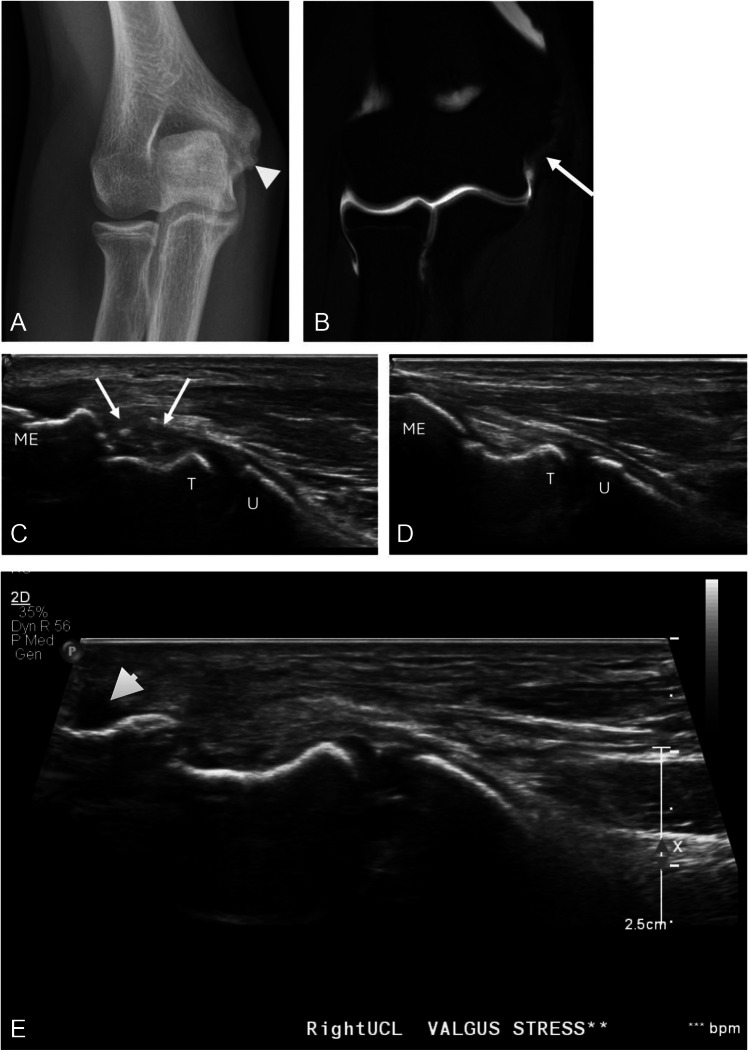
A 14-year-old male baseball pitcher presented with recurrent right elbow pain 1 year after surgical fixation of a medial epicondylar avulsion fracture. A Coronal radiograph demonstrates osseous remodeling of the medial epicondyle without evidence of an acute avulsion. B Coronal T1 fat-saturated MR arthrogram image shows a heterogeneous, thickened proximal UCL without a discrete tear (*arrow*). C Stress ultrasound of the elbow, performed at the surgeon’s request, again demonstrates osseous remodeling of the medial epicondyle. The proximal ulnar collateral ligament appears thickened with internal echogenic sutures but without a definite tear. D US of the normal contralateral elbow for comparison. No asymmetric increase in ulnohumeral joint gapping during valgus stress maneuvers. E Cine clip obtained during dynamic stress evaluation reveals a small focal partial tear of the common flexor tendon attachment (*arrowhead*)
